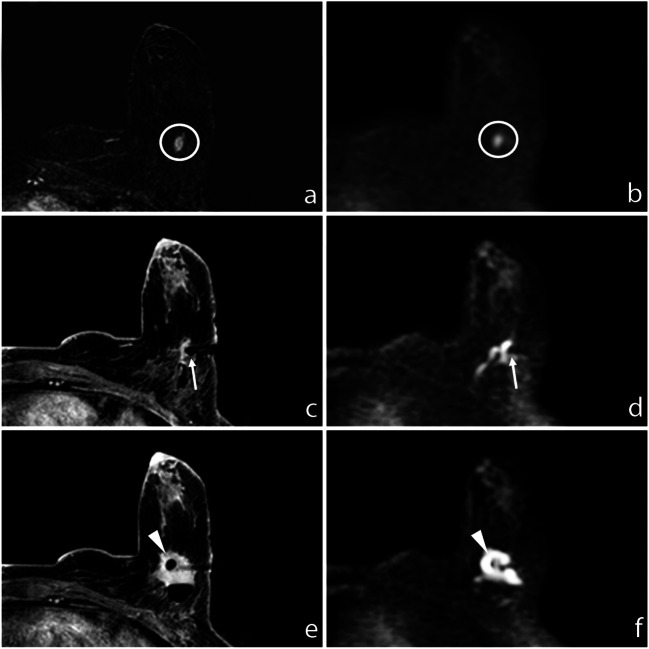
a Subtracted DCE 3D-THRIVE (T1-weighted high-resolution isotropic volume excitation) showing a mass lesion (circle) in the deep equatorial plane of the left breast of a 75-year-old woman. b DWI (*b* = 1300 s/mm^2^) showing hyperintensity of the enhancing lesion (circle). c T1-WI FS GRE showing the proper position of the biopsy device (arrow); the end of the needle is recognizable at (d) DWI (*b* = 1300 s/mm^2^, arrow). e T1-WI FS GRE showing the correct deployment of the site marker that is also recognizable at (f) final DWI (*b* = 1300 s/mm^2^, arrowhead)
